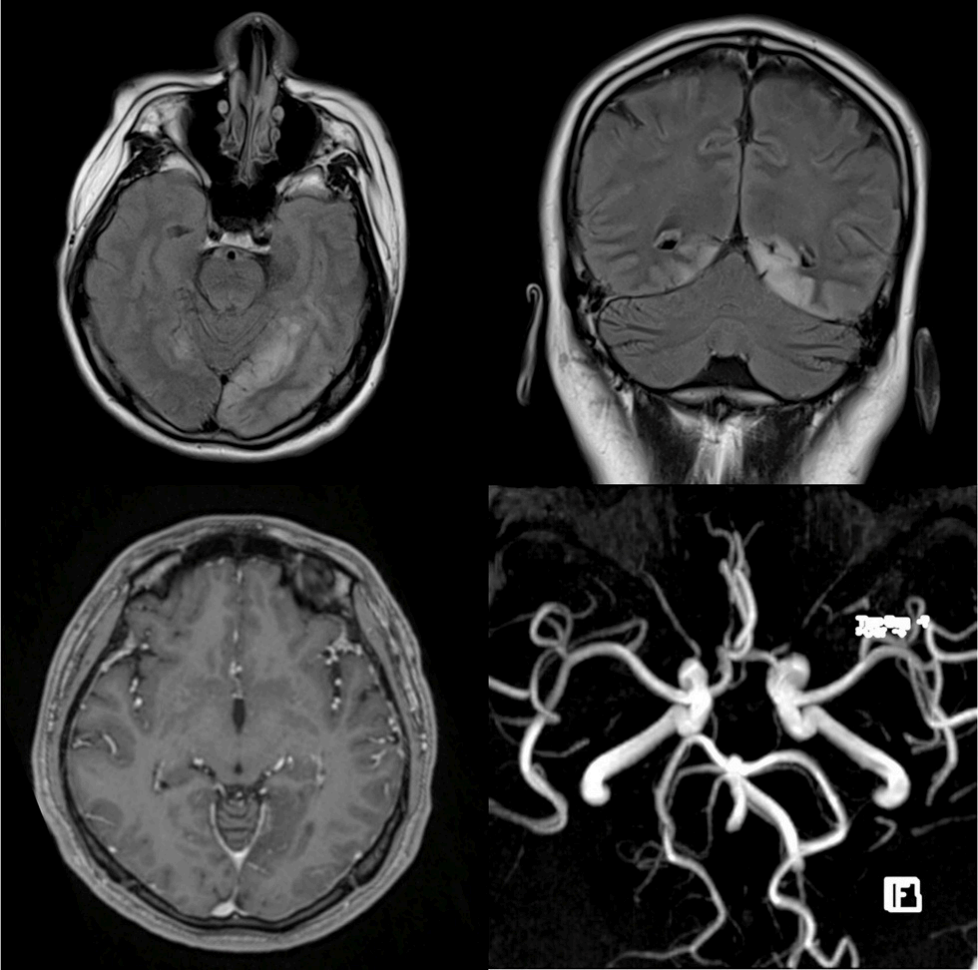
T2-weighted FLAIR MRI images on the axial (top left) and coronal (top right) planes exhibit abnormal hyperintense signals involving the cortices of both occipital lobes consistent with encephalitis. T1-weighted post gadolinium shows no enhancement of the affected occipital lobe (bottom left) and MR angiogram (MRA) is normal with no evidence of vasculitic changes (bottom right).
